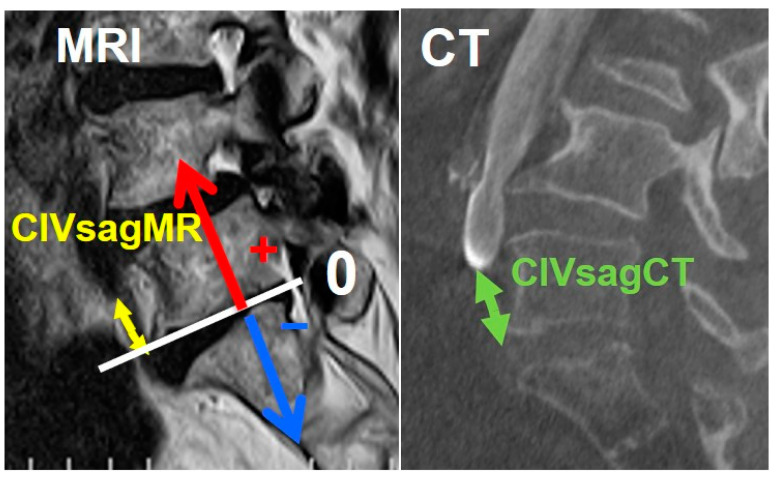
Measurement of common iliac bifurcation either on MRI or CT. The position was measured from the L5 caudal endplate to the bifurcation and expressed as plus (cephalad) or minus (caudal). CIVsagMR: common iliac vein in sagittal MRI; CIVsagCT: common iliac vein in sagittal CT.
